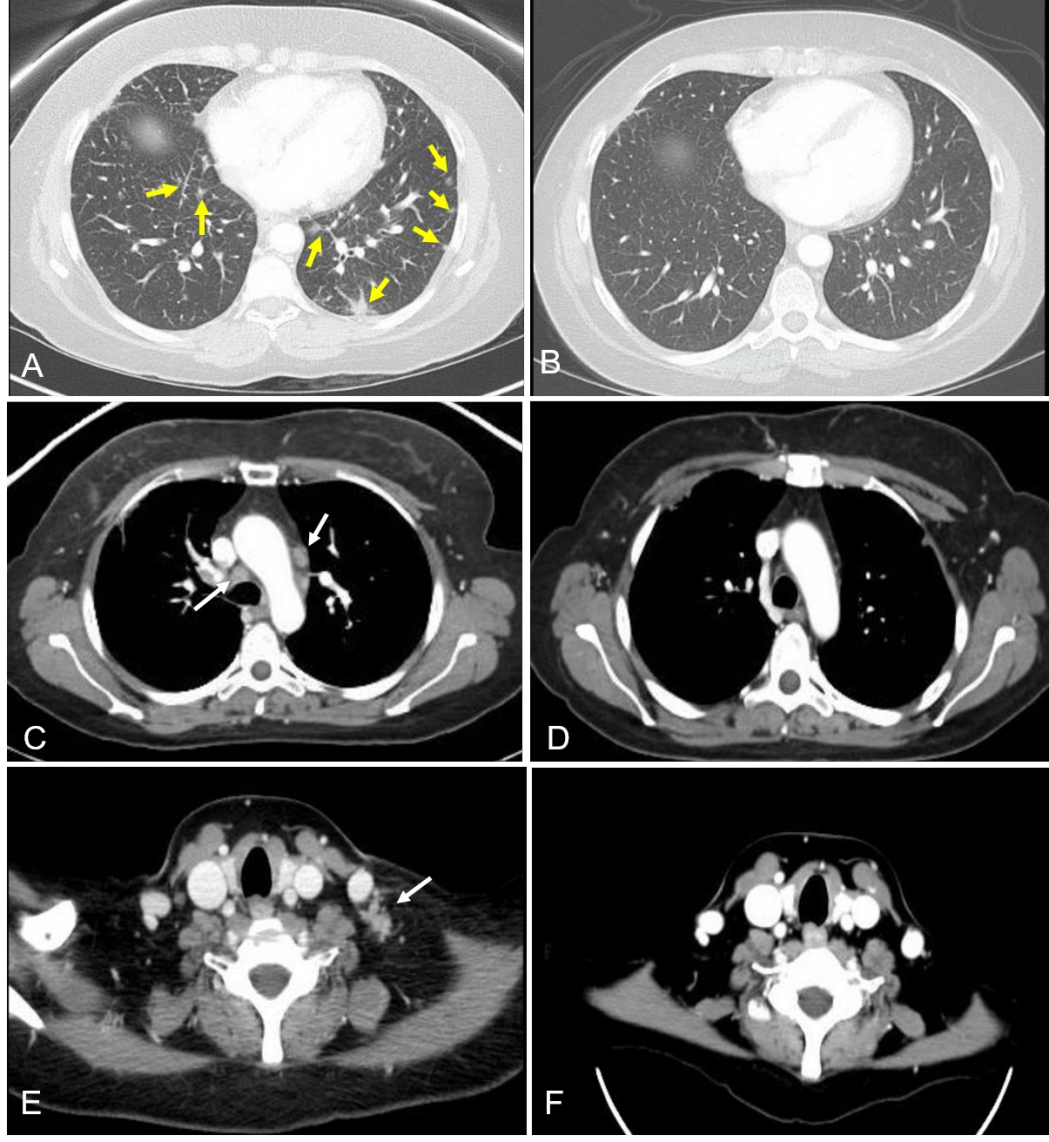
Comparison of computed tomography images of lung and neck before and after treatment Pulmonary lymphangitic carcinomatosis manifested with numerous, ill-defined, bilateral peribronchovascular nodular opacities; (A) yellow arrows pointing at the nodular thickening of interlobular septa and several lateral and posterior nodules. A follow-up computed tomography scan after three cycles of ado-trastuzumab emtansine treatment showed resolution of most nodules (B). Marked mediastinal (C) and cervical (E) lymphadenopathy before treatment is shown (arrows). A follow-up computed tomography study after treatment showed completely resolved lymphadenopathy (D, F).
